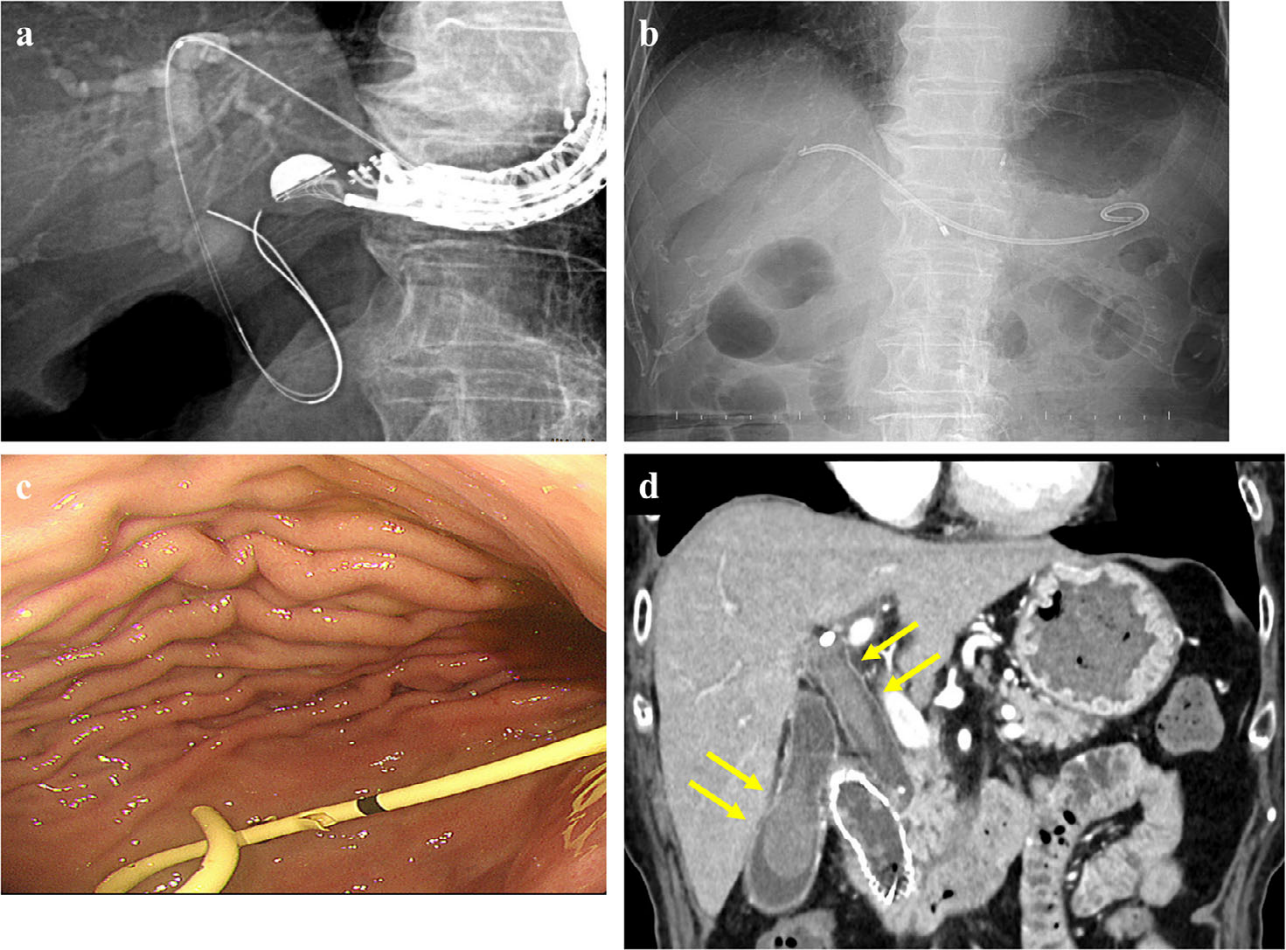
X‐ray and endoscopic images of endoscopic ultrasound‐guided hepaticogastrostomy (EUS‐HGS) performed before chemotherapy administration and Contrast‐enhanced tomography images of biliary bleeding on day 46 after EUS‐HGS. (a, b) A 7‐Fr plastic stent for EUS‐HGS (Type IT Stent) was placed with the hepatic side end of the plastic stent at the hepatic hilum via B3. (c) The stomach end of the plastic stent was placed at the lesser curvature of the lower body of the stomach, and bile flowed out through the plastic stent. (d) On day 46 after EUS‐HGS, Biliary bleeding, which was visualized as a high‐density area, was observed in the common bile duct and gallbladder (yellow arrow).
